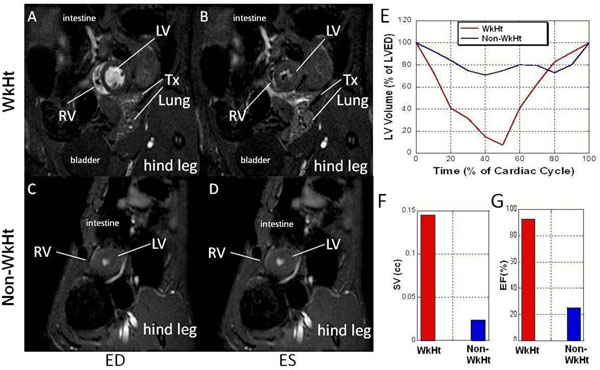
Cardiac functions of isograft hearts from 2 HTx models evaluated by cine MRI. (A, B) cine imaging of an isograft from the WkHt model; (C, D) cine imaging of an isograft from the Non-WkHt model; (A, C) cine images acquired at end-diastole (ED); (B, D) cine images acquired at end-systole (ES); (E) temporal dynamic of normalized left ventricular volume over a cardiac cycle, (F) SV, and (G) EF, of a WkHt (red) and a Non-WkHt (blue).
